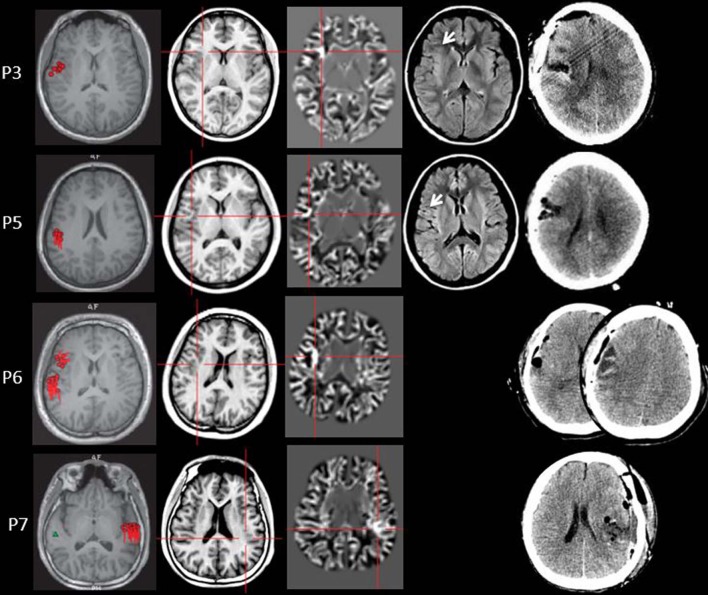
The MEG, MAP, and post-surgical results of patients with positive MAP results (Patient 3, 5, 6, and 7). The crosshairs pinpoint the location of the MAP-positive region. First column: MEG results of Patient 3, 5, 6, and 7. Second column: T1-weighted magnetization prepared rapid acquisition with gradient echo (MPRAGE) images used as input to MAP. Third column: gray–white matter junction z-score file as the output of MAP. Fourth column: T2-weighted fluid-attenuated inversion recovery (FLAIR) images of P3 and P5; the FLAIR images of P6 and P7 were not available. Fifth column: post-operative CT scan indicating site and extent of resection. Three patients with positive MAP results (Patient 3, 5, and 7) had complete resection of the MAP-positive regions, and remained seizure-free at 12 months after surgery. One patient (Patient 6) had incomplete resection of the MAP-positive regions and this patient still had seizure at 12-month follow-up. Pathology: P3, FCD IIb; P5, FCD IIa; P6, FCD IIa; P7, FCD IIb.
